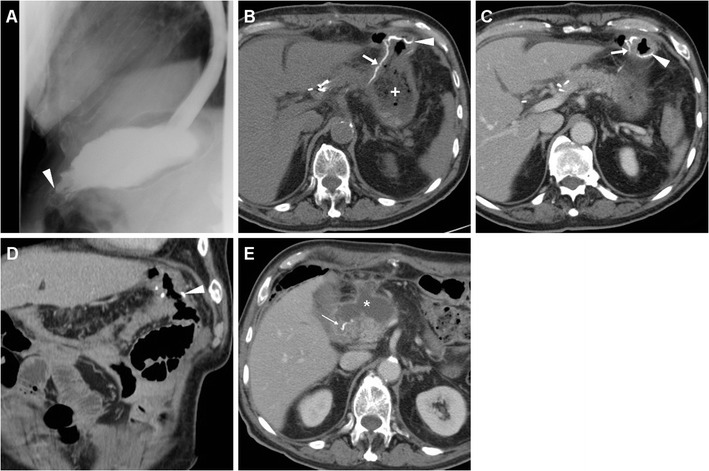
A 78-year-old male with antral gastric cancer underwent subtotal gastrectomy with D2 lymphadenectomy and Billroth II reconstruction and suffered from postoperative vomiting and fever. On 5th POD CF (A) showed limited, slow transit through the GJS (arrowhead). On 9th POD, unenhanced (B) and post-contrast (C-E) CT showed stapled gastric resection site (arrows) and GJS (arrowheads), minimally dilated remnant stomach (+), and a fluid collection (* in E) abutting the DS (thin arrow) consistent with DS leakage, which was successfully managed conservatively but required prolonged hospitalization
